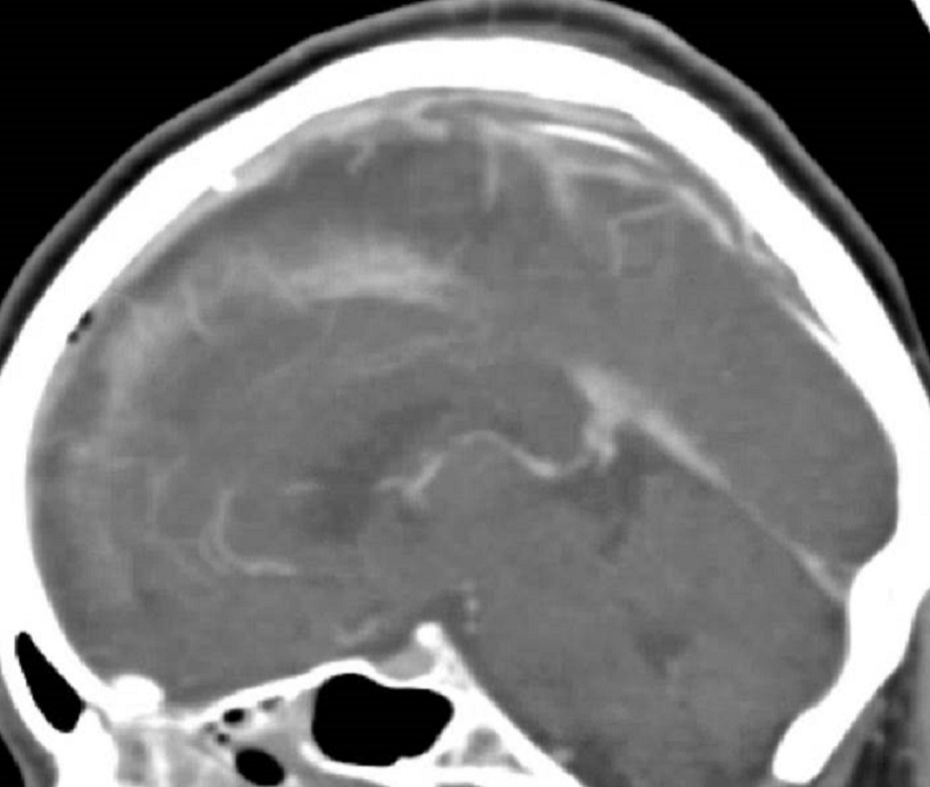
Contrasted CTA 10 hours Post-Infusion CONTRASTED CTA obtained after 10-hour infusion reveals flow through the sagittal and straight sinus, the vein of galen, and the internal cerebral veins. The catheter tip and non-occlusive thrombus in the sinus are visible.
